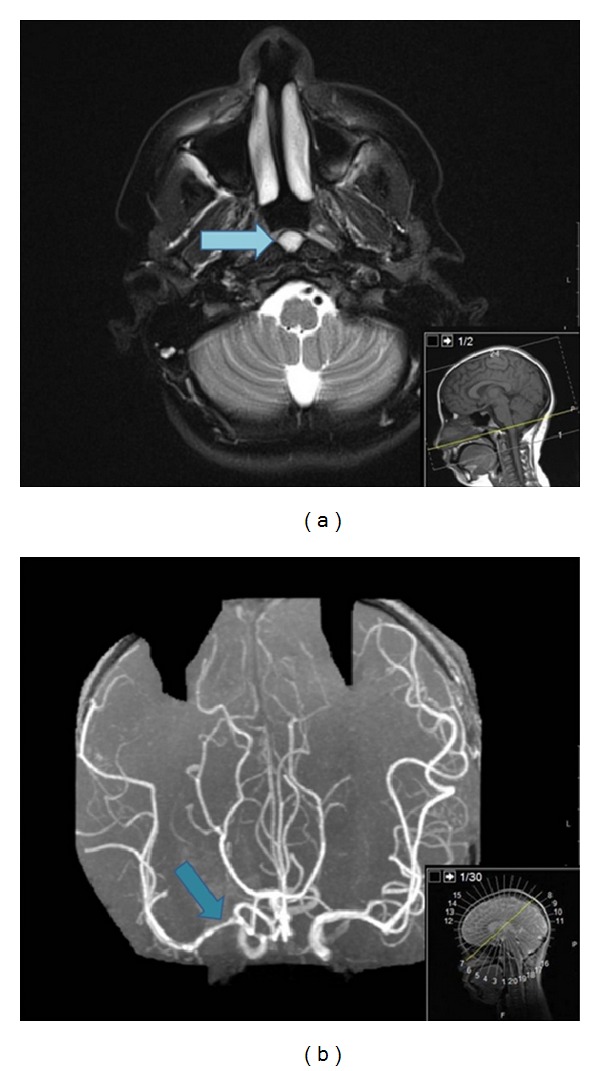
Magnetic resonance images from patient depicting abnormal findings. (a) Axial T2 weighted MR image through the nasopharynx demonstrating a small well-circumscribed T2 hyperintense, CSF-like cyst nestled between the longus colli muscles deep to the nasopharyngeal mucosa consistent with an uncomplicated Tornwaldt's cyst (arrow). (b) 3D time-of-flight MRA of the intracranial circulation demonstrating narrowing and irregularities with the wall of the M1 segment of the right middle cerebral artery (arrow) indicating, but not limited to, arteritis or dissection.
